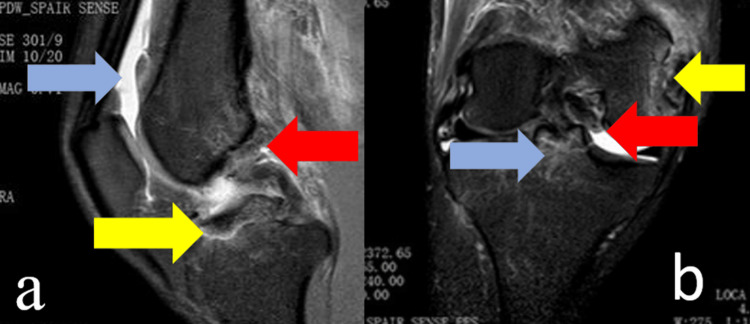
Magnetic resonance imaging (MRI) of the right knee. (a) Sagittal view demonstrates avulsion of the anterior cruciate ligament (ACL) from the tibial insertion (yellow arrow), posterior cruciate ligament (PCL) avulsion from the femoral attachment (red arrow), and joint capsular effusion (blue arrow).
